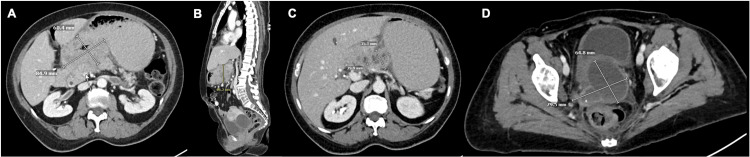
Post-chemotherapy imaging demonstrating marked disease progression (A) Axial contrast-enhanced CT of the abdomen showing significant interval enlargement of the distal gastric mass (8.5 × 6.0 cm; previously 7.0 × 3.0 cm), now confluent with enlarged gastrohepatic nodal metastases. (B) Sagittal contrast-enhanced CT of the abdomen demonstrating loss of the fat plane between the gastric mass and pancreas, concerning for direct invasion. (C) Axial contrast-enhanced CT of the abdomen showing marked interval enlargement of gastrohepatic lymph node metastases. (D) Axial contrast-enhanced CT of the pelvis demonstrating interval growth of the pelvic mass involving the uterine cervix. These images were obtained at a later imaging time point than those shown in Figures 2-3.
